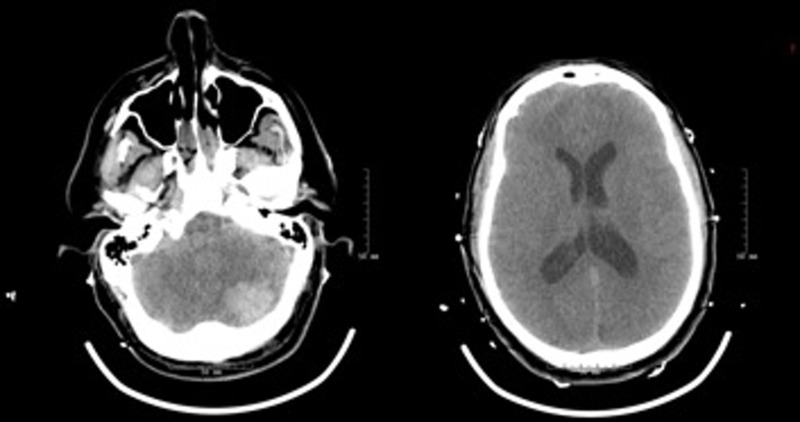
Interval computed tomography (CT) demonstrating enlarging cerebellar hemorrhage and dilated lateral ventricles
